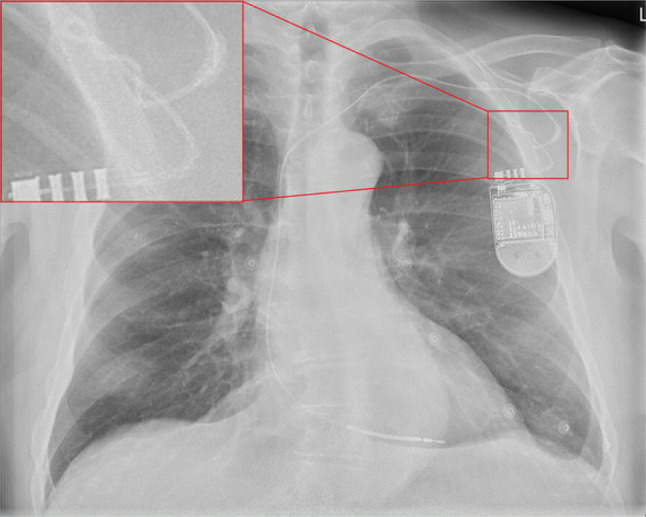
Same chest radiograph as shown in Fig. 1 in the question, with enlargement of proximal part of ventricular lead of the implantable cardioverter-defibrillator
